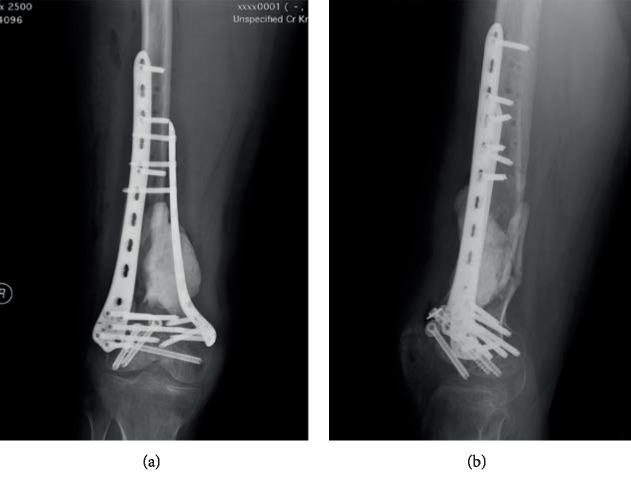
Postoperative radiograph of the right knee. Anteroposterior (a) and lateral (b) radiograph of the right knee shows the revision of internal fixation and cement placement in the bone defect.
